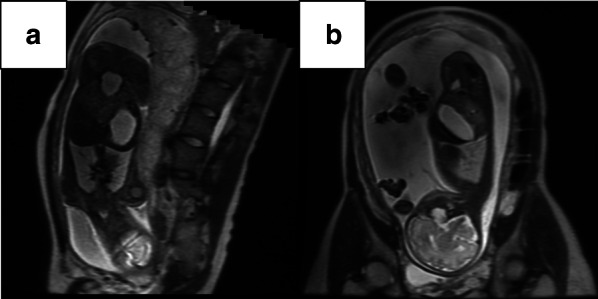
Fetal MRI showing polyhydramnios and dilated stomach in Case 2: a axial view, b sagittal view
